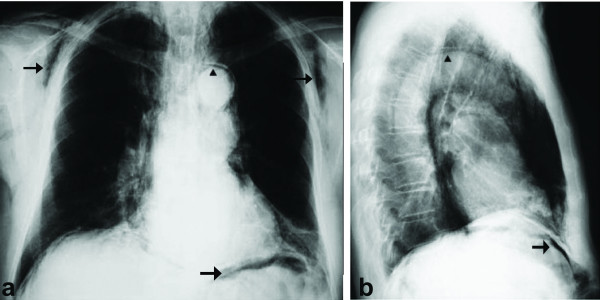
Chest radiographs revealing the presence of subcutaneous emphysema, pneumomediastinum and pneumoperitoneum (arrows).
